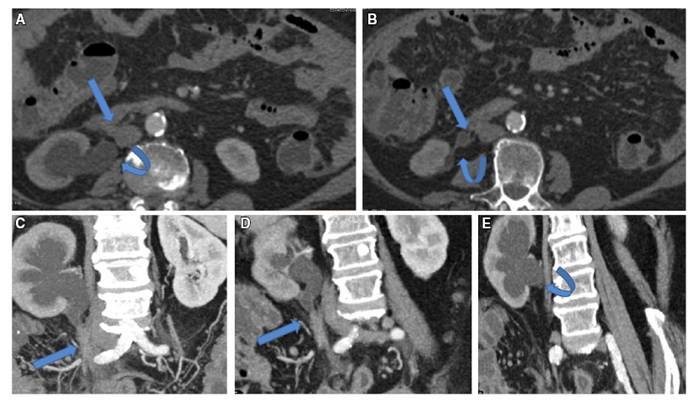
CT (A&B) axial, (C-E) Coronal MPR showing: AIP patient showing right retroperitoneal fibrotic band (Arrows), entrapping and compromising the upper right ureter, with secondary right upper hydroureter and hydronephrosis (Curved arrows).
